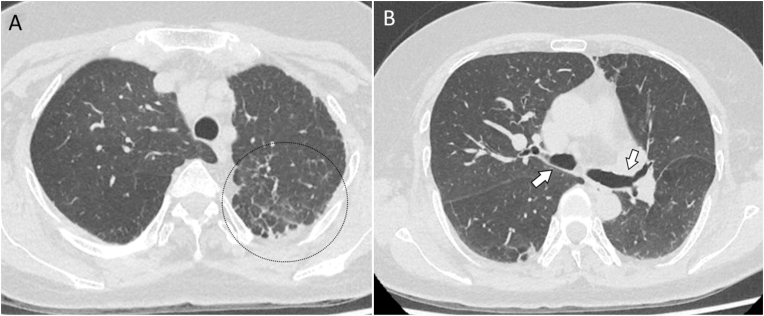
High-Resolution CT Demonstrating Limited Parenchymal Disease and Bronchial Displacement High-resolution computed tomography (lung window). (A) Focal interstitial abnormalities in the apical segment of the left upper lobe (dotted circle), insufficient to explain the severity of pulmonary hypertension. (B) Posterior displacement of the left main bronchus (right arrow) secondary to extrinsic compression from the tortuous left pulmonary artery (LPA). The right main bronchus (left arrow) follows a preserved anatomical course. These findings support a predominantly vascular rather than parenchymal mechanism.
